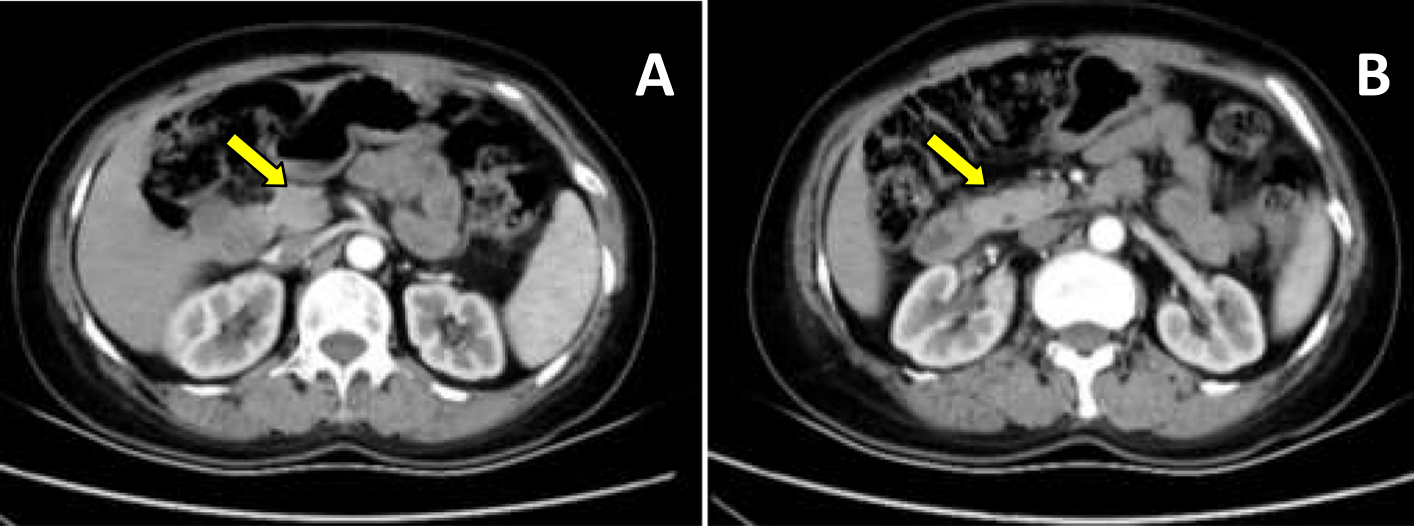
a and b Axial arterial phase CT image showing the absence of the pancreatic body and tail, without pancreatitis and pancreatic duct dilatation. The *arrow* show the pancreatic head
